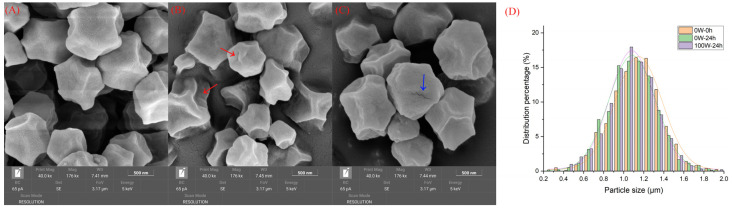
Effects of ultrasonic treatment on the microstructures ^1^ ((A) 0 W–0 h; (B) 0 W–24 h; and (C) 100 W–24 h) and particle size distributions (D) of starch samples extracted from quinoa samples under different treatments during germination. The image magnification was approximately 40 k× for whole granules. ^1^ The red and blue arrows were used to point to crimples and fissures, respectively.
